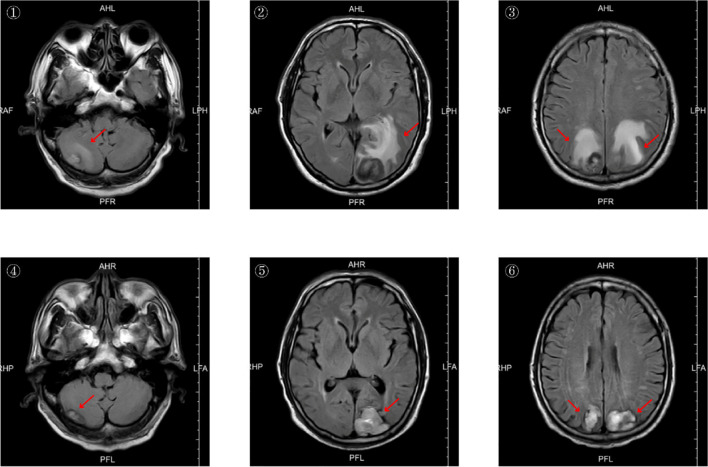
Pre- and post-treatment cranial MRI scans. Figures ①②③ show pre-treatment cranial MRI on July 16, 2025, revealing bilateral parieto-occipital and right cerebellar masses accompanied by significant edema. Figures ④⑤⑥ show cranial MRI scans taken on October 30, 2025, after treatment. The original edema has resolved, and the cranial metastatic tumors have also decreased in size compared to the previous scans.
